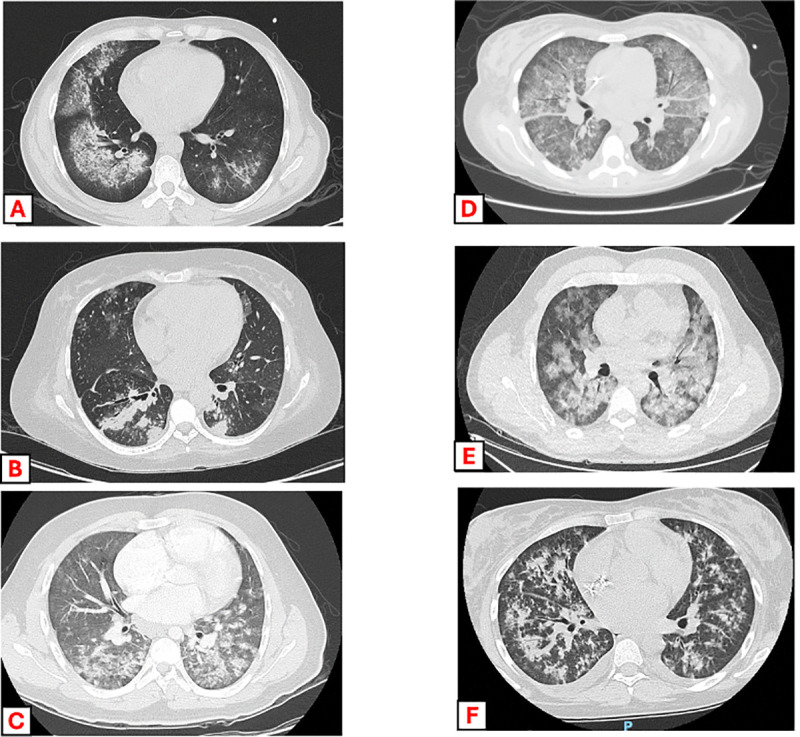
Chest CT findings in patients with diffuse alveolar hemorrhage, with and without pulmonary infections. (A–C) Cases with pulmonary infection: chest CT scans demonstrate bilateral ground-glass opacities and consolidations with superimposed interlobular septal thickening, patchy alveolar infiltrates, and areas of consolidation predominantly in the lower lobes, consistent with alveolar hemorrhage associated with coinfection. (D–F) Cases without pulmonary infection: chest CT scans reveal extensive bilateral ground-glass opacities and diffuse alveolar consolidation with a “crazy-paving” pattern, but without focal findings suggestive of superimposed infection.
